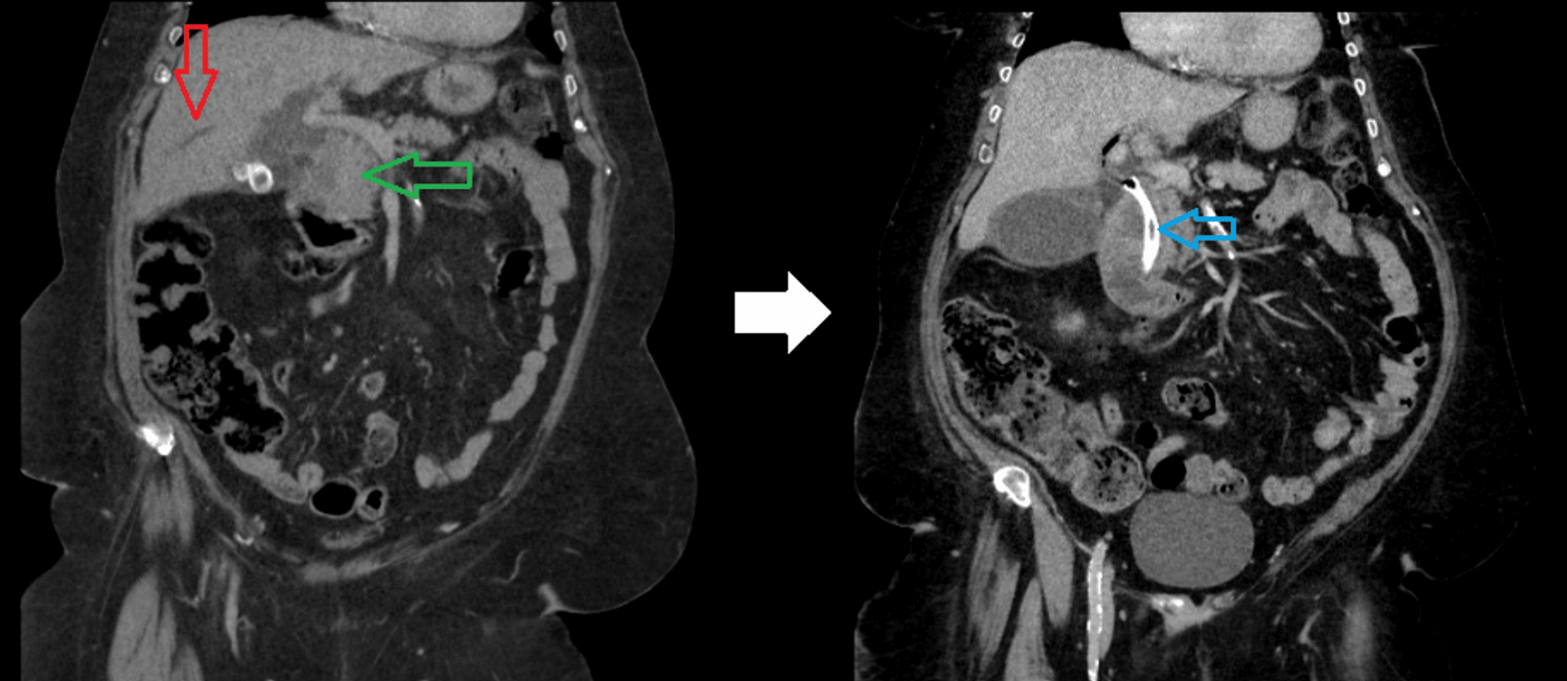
Cholangiocarcinoma imaging The left image shows a coronal view of a CT of the abdomen/pelvis. There is intrahepatic dilation (red arrow) as a result of an ampullary/pancreatic head lesion (green arrow). The image to the right of the white arrow is another coronal view of a CT of the abdomen/pelvis taken approximately a week later. It shows the placement of a common bile duct stent (blue arrow). CT: computed tomography
